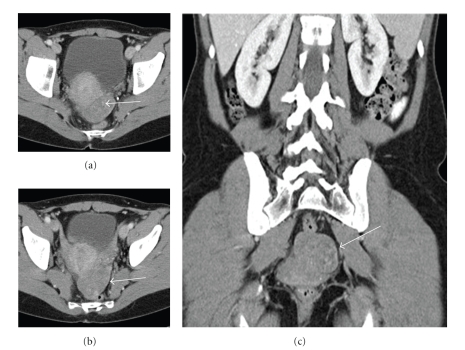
CT scan of the abdomen and pelvis (1/12/208). Axial (a) and (b) and coronal (c) images of the pelvis demonstrate a large, predominantly hypodense, left adnexal soft tissue mass (white arrow). Note that the cystic component of the mass has resolved and the soft tissue portion has significantly increased.
